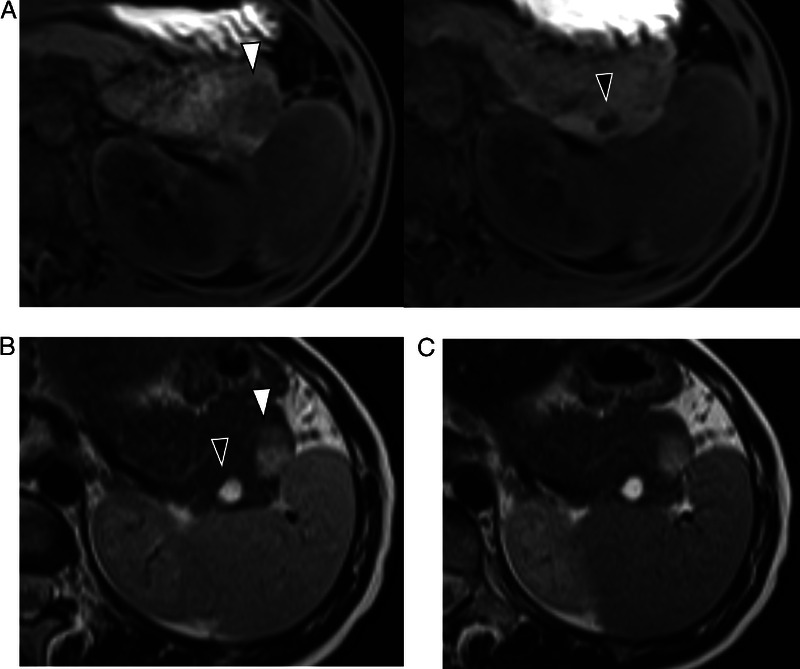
Magnetic resonance imaging. A, A T1-weighted imaging revealed areas of slight hypointensity. B, A T2-weighted imaging revealed areas of slight hyperintensity. C, The peripheral portion of the cysts had no areas of hypointensity like that of the spleen on superparamagnetic iron oxide imaging.
